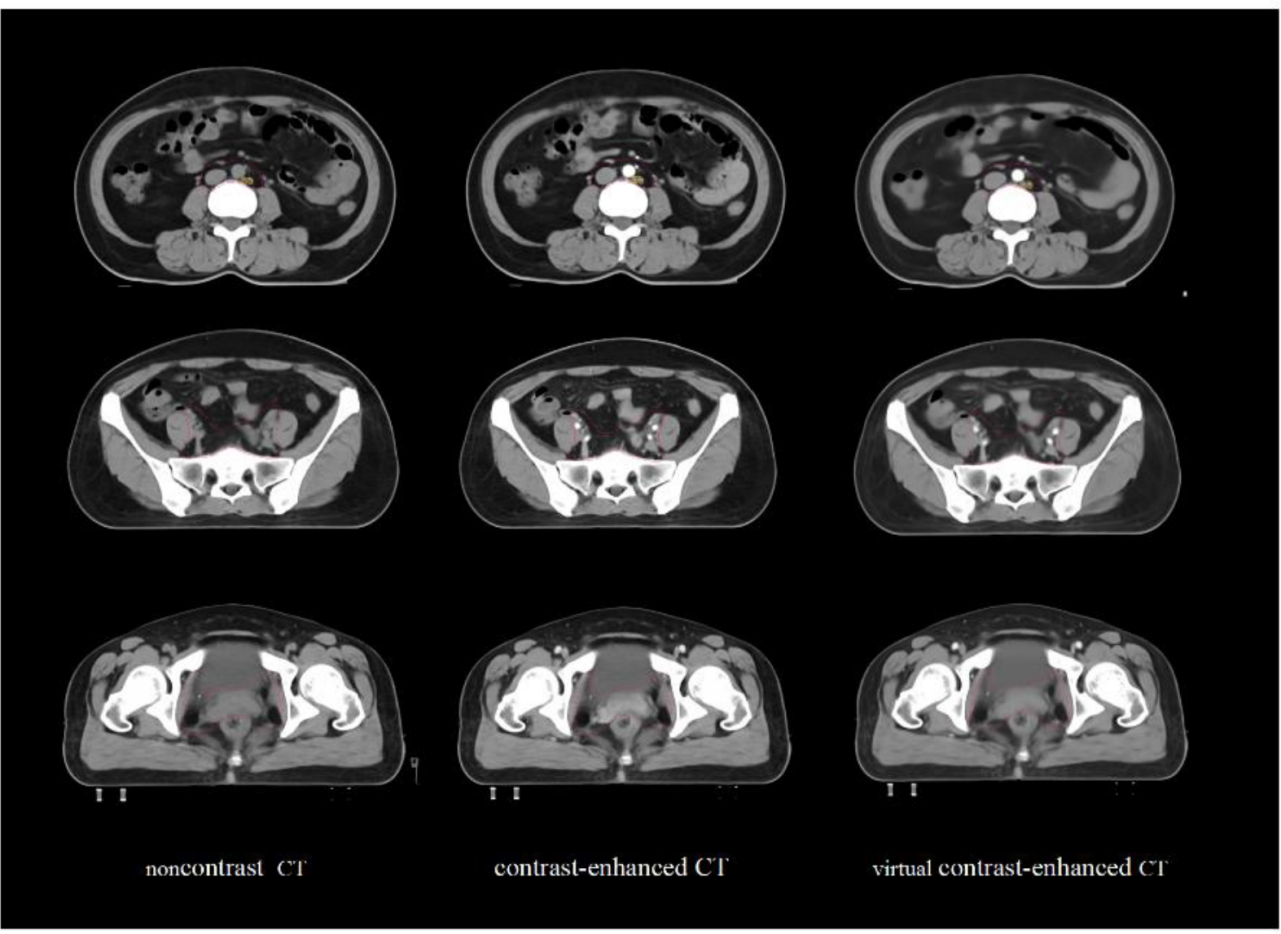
Comparison of noncontrast CT, contrast-enhanced CT and virtual contrast-enhanced CT images in the same slice.
